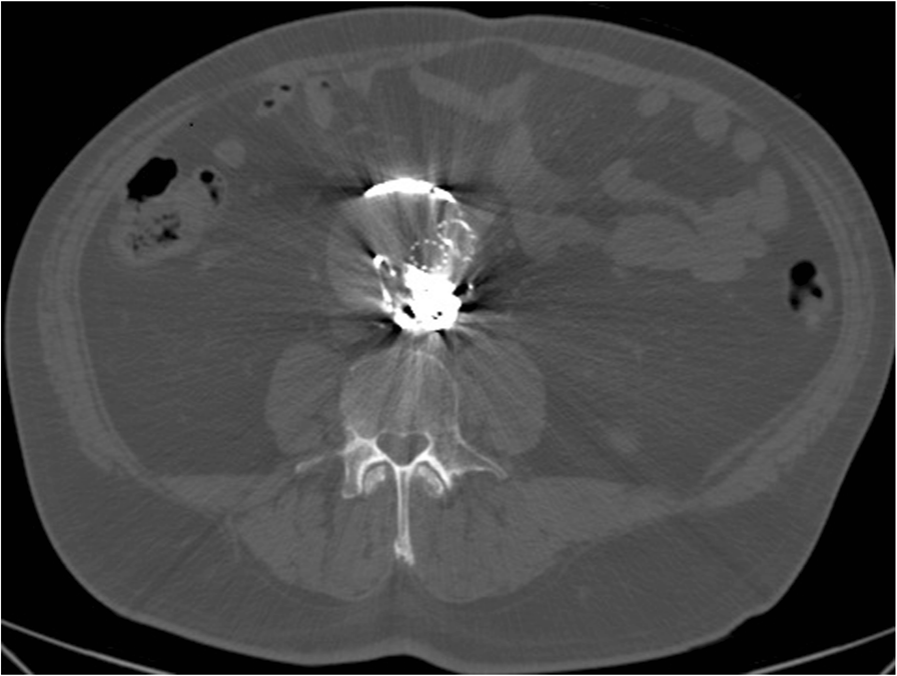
Post-embolization axial CTA through the lower abdomen at the same level as image in Fig. 1 shows embolic material filling the portion of the aneurysmal sac which previously demonstrated endoleak
